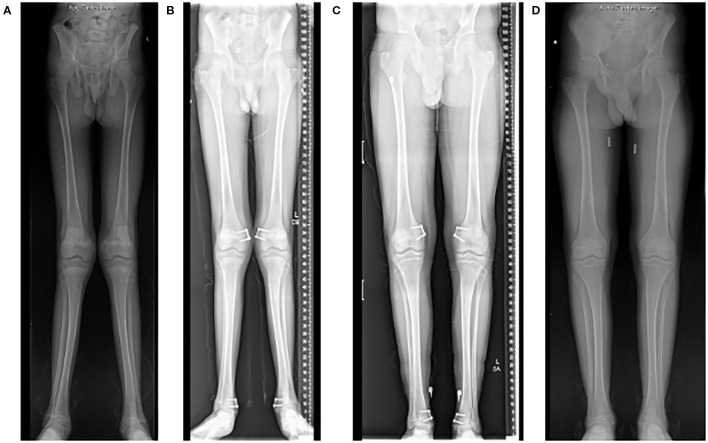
Radiographs of a 15-year-old who presented with significant genu valgum (“knocked knees”) (A). He underwent a guided-growth procedure using tension band plates on the medial side of both his distal femur and distal tibia physes (B). The hardware was removed 2 years after the procedure once satisfactory deformity correction was achieved (C, D).
